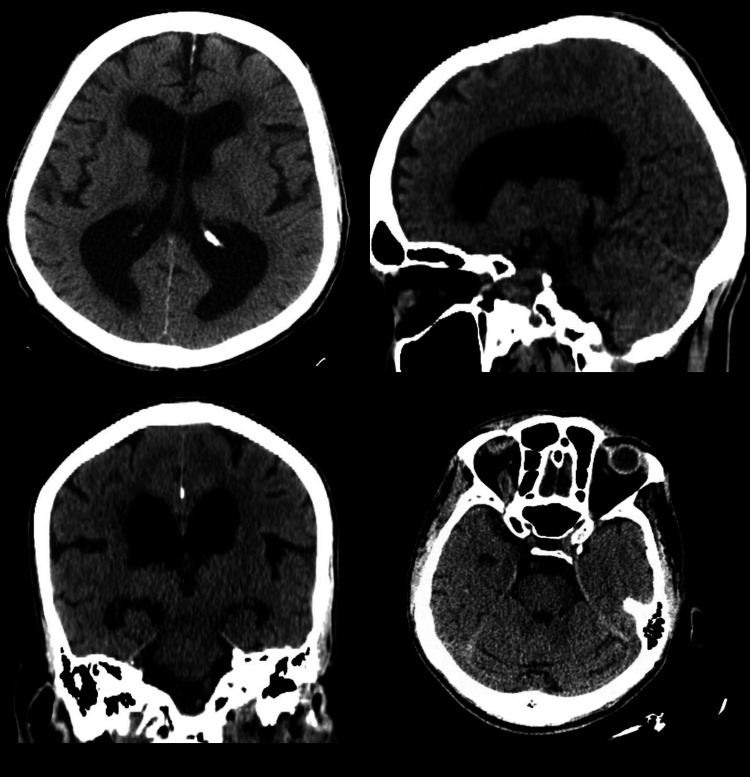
CT scan of the head showing no acute intracranial pathology CT: computed tomography
